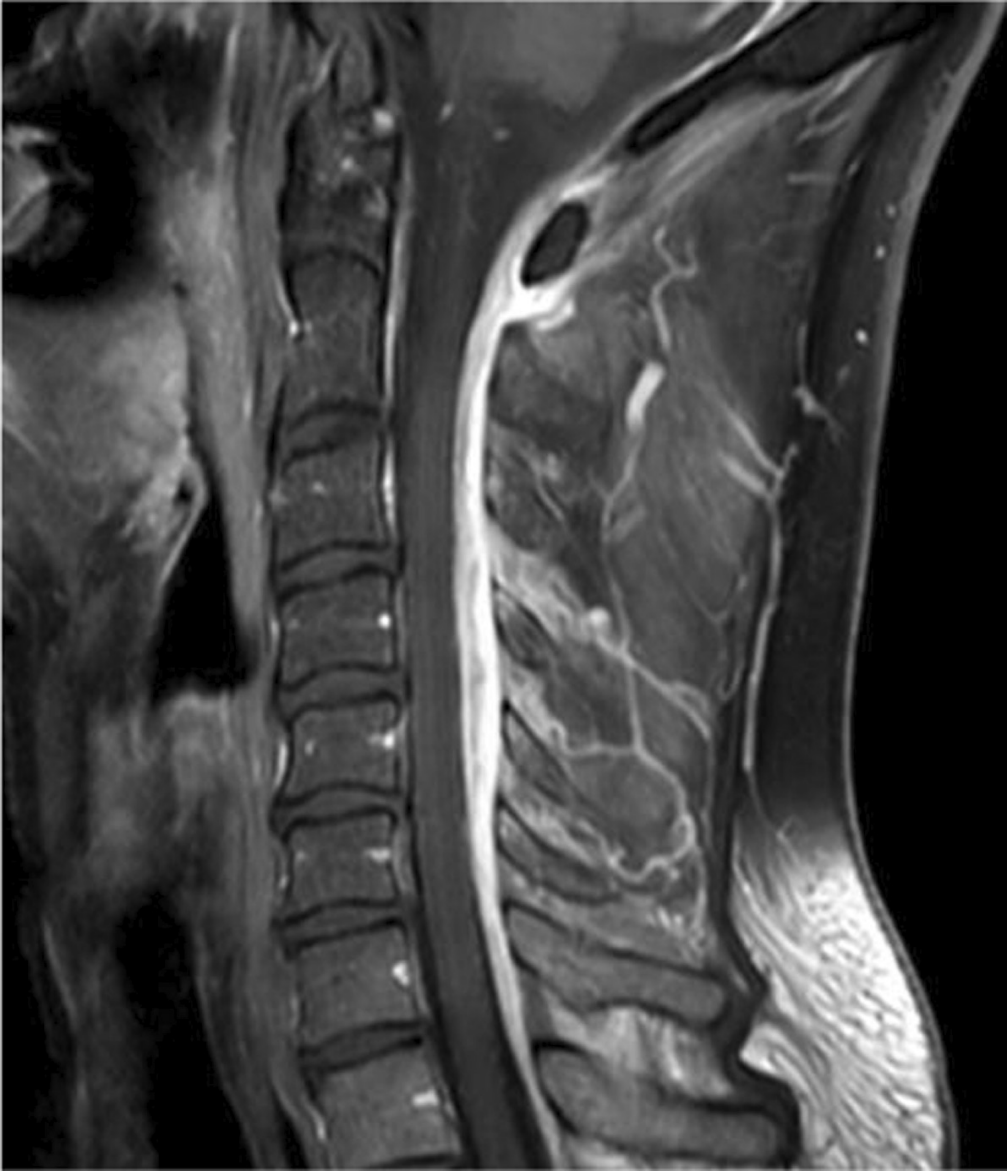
MRI scan in T1 sequence with gadolinium contrast agent showing dorsal epidural enhancement consistent with an epidural abscess
